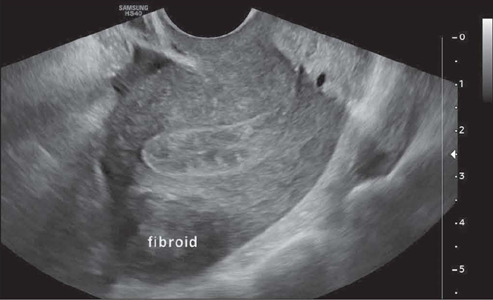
Transvaginal ultrasound image, in a longitudinal view, showing a subserosal fibroid
